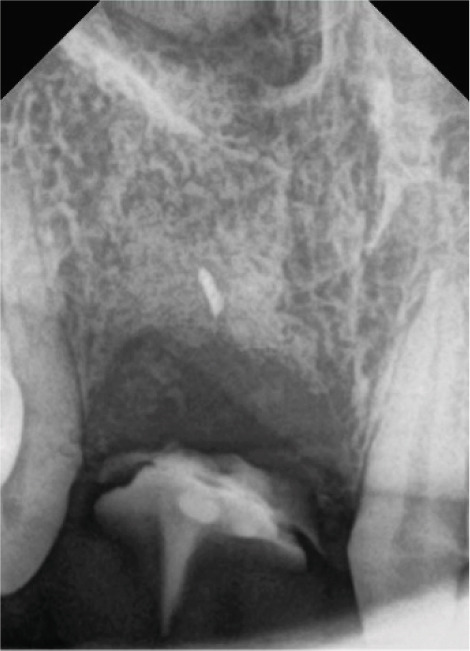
Periapical radiograph before treatment.
